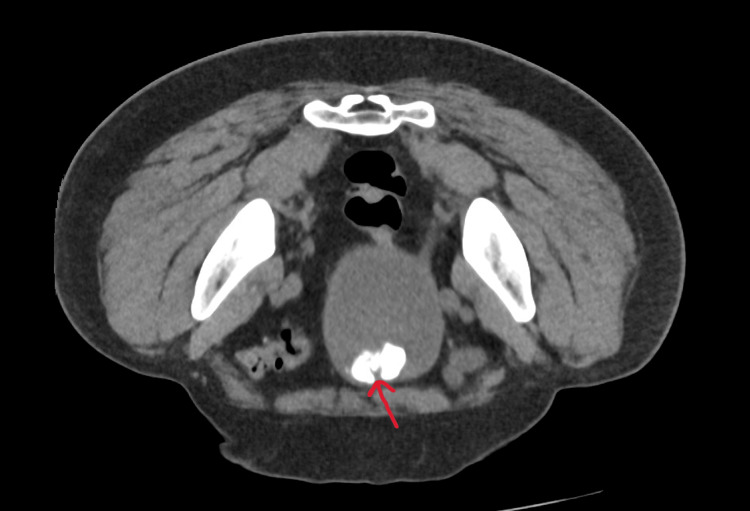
Computed tomography of the kidneys, ureters and bladder showing a large calcification in the bladder (red arrow).
